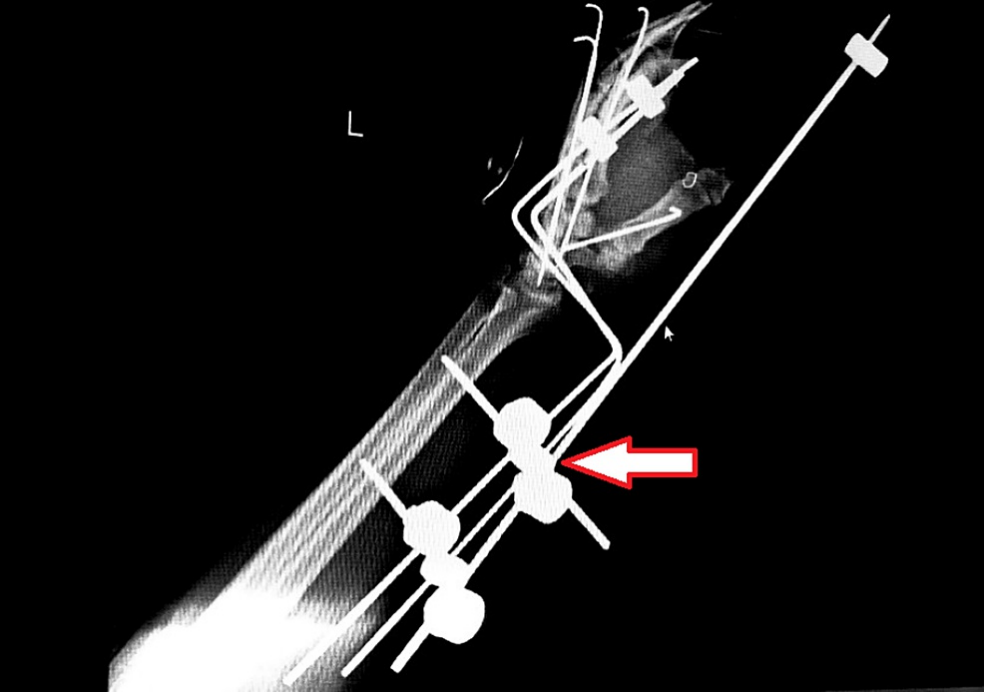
Post-operative X-ray of the left hand The implant seen is a JESS ( Joshi's External Stabilization system) fixator
